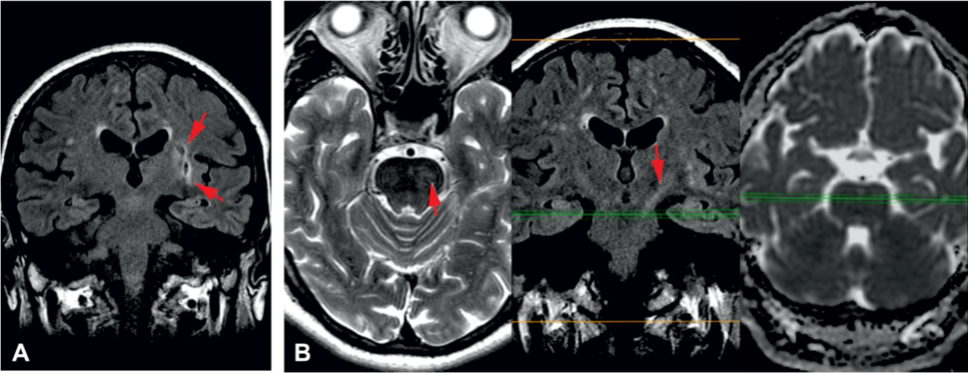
A, B Polygonal left side area intra- and supranuclear hyperintense T2, hypointense with hyperintense periphery on FLAIR images, which interface pyramidal fibers in the posterior arm of internal capsule leeding to Wallerian degeneration of them - left lateral pontine dots that apare hyperintense on T2 and FLAIR images, without restricted diffusion
